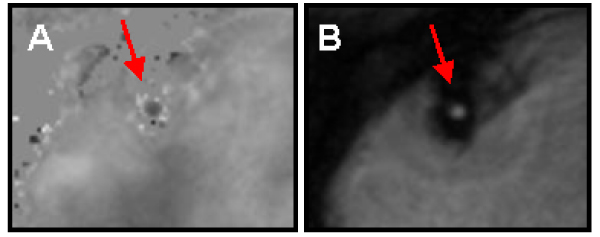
Cross-sectional images of the RCA at rest in a subject using velocity-encoded MRI. (A) Phase image and accompanying (B) Magnitude image.
